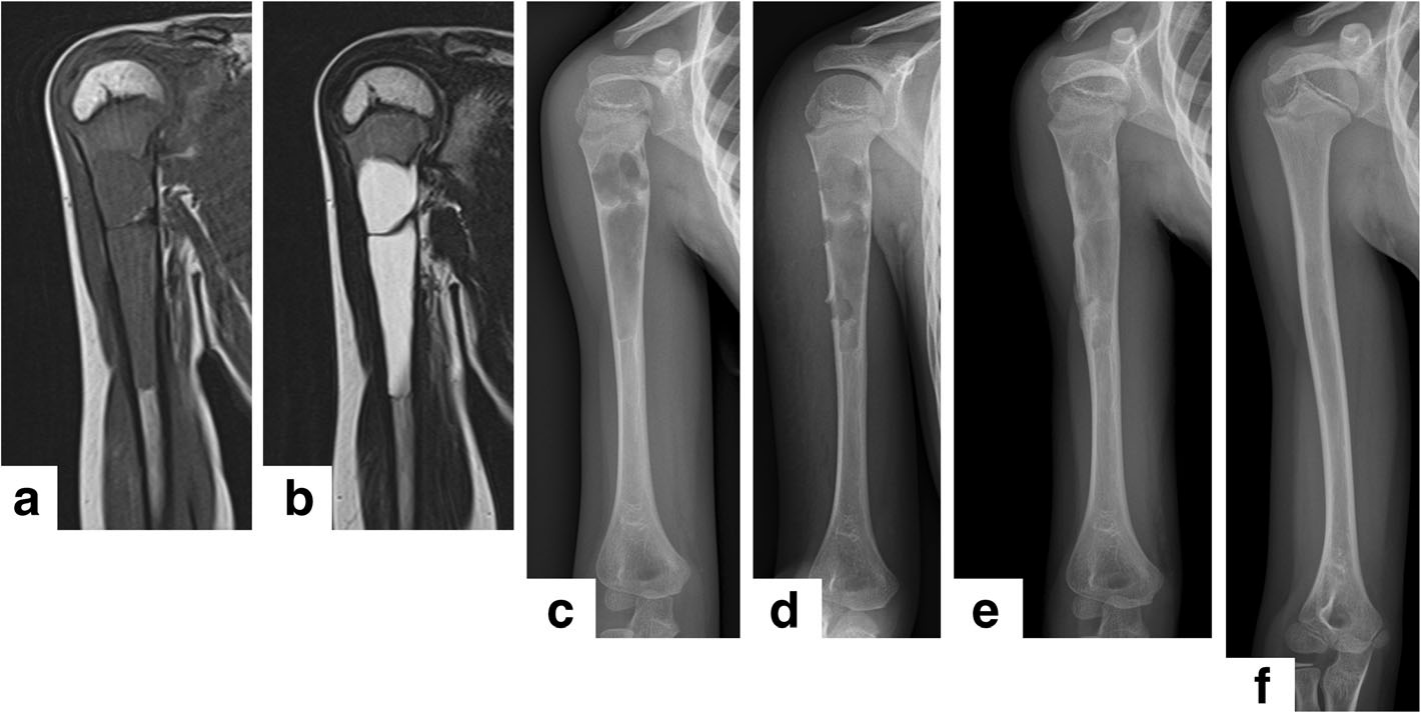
The typical case of simple bone cyst in the right humerus treated with endoscopic curettage. A 6-year-old boy bruised his shoulder (a T1-weighted magnetic resonance [MRI]; b T2-weighted MRI; c X-ray). After 6 months of conservative therapy, the patient underwent endoscopic curettage via three portals (d postoperative image). Three months after the procedure, healing was confirmed with a callus around the portals and consolidation in the cavity (e solid union). Three years later, the bone was remodeled without any residual tumor or angular deformity (f class A)
